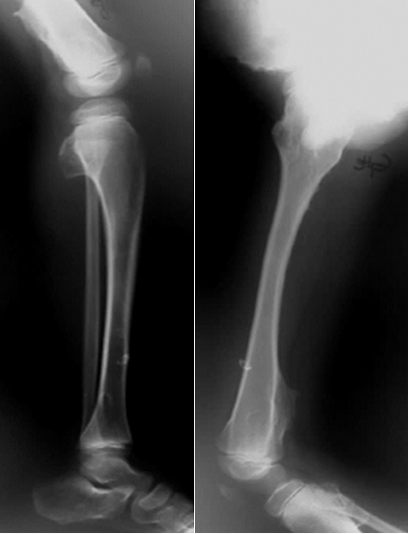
Radiographie des MI, formations osseuses xérophytiques en continuité avec le cortex de la partie proximal du tibia à gauche et de la région métaphysaire inférieure du fémur: exostose
